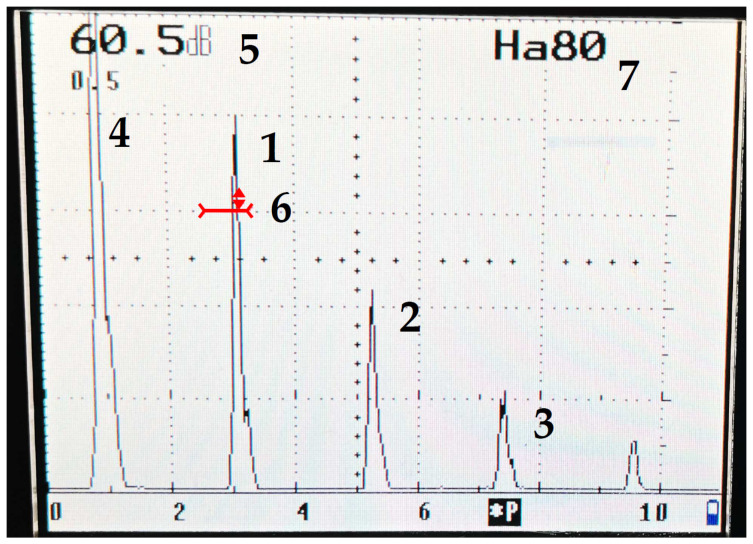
Ultrasonic flaw detector; 1—first pulse (H_I_), 2—second pulse (H_II_), 3—third pulse (H_III_), 4 —transmit impulse, 5—gain, 6 —gate (red color), 7—amplitude value of gate pulse.
